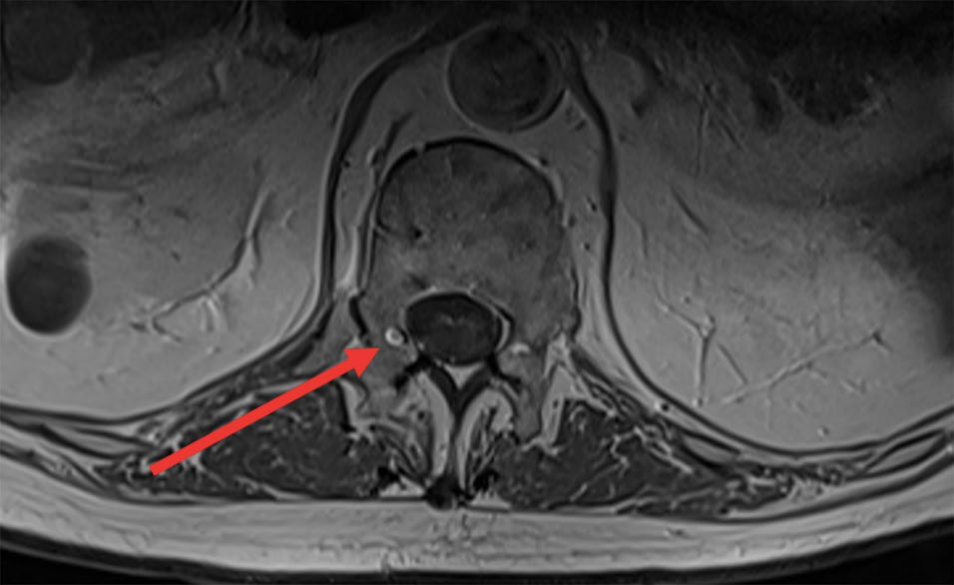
MRI of spinal cord T1 (+ gadolinium) sequence, showing a 2-mm abscess with a “ring enhancement” in right posterolateral area of the spinal cord in D12-L1 (arrow)
